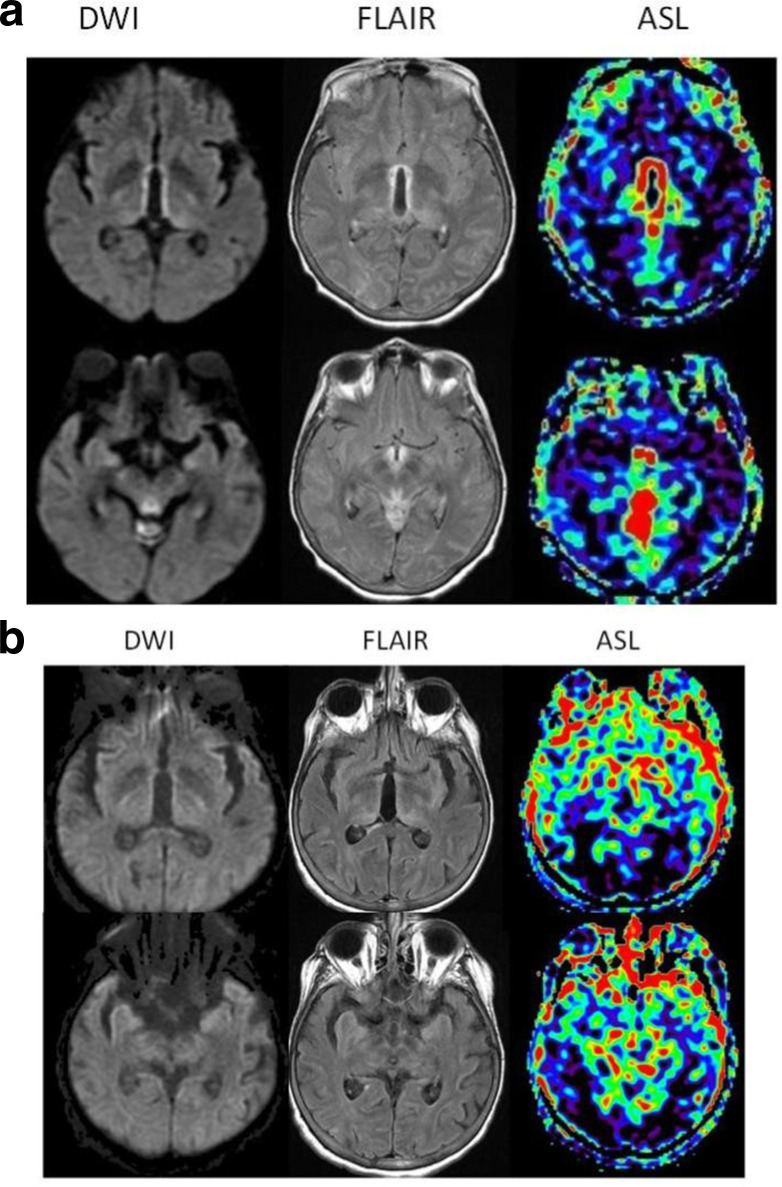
(a) Pre-treatment MRI of Patient 1 demonstrates restricted diffusion and T2 prolongation along the classically involved regions of the medial thalami, mammillothalamic tracts, mammillary bodies, and periaquectal gray. These lesions correspond to increased CBF on ASL, with diffuse cerebral cortical hypoperfusion. (b): Post-treatment MRI of Patient 1 demonstrates improvement of restricted diffusion and T2 prolongation along the medial thalami, mammillothalamic tracts, mammillary bodies, and periaquectal gray. There is corresponding normalization of CBF in these regions, as well as improvement of previously seen cortical hypoperfusion.
